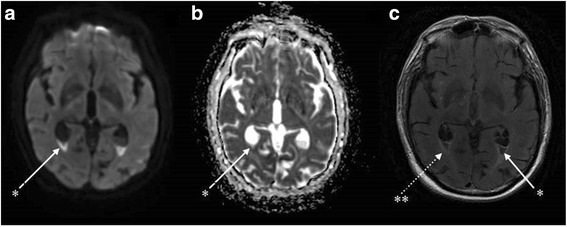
Brain magnetic resonance imaging (day 6) showing an aspect of ventriculitis in multiple axial sequences. *Irregular declivous intraventricular debris with restricted diffusion in the occipital horns with a moderate dilatation of the lateral ventricles seen in all sequences: diffusion (a), apparent diffusion coefficient (b) and T2 fluid attenuated inversion recovery (FLAIR) gadolinium (1c). Periventricular hyperintensities and ependymal enhancement in the axial T2 FLAIR with gadolinium enhancement sequence (c)
